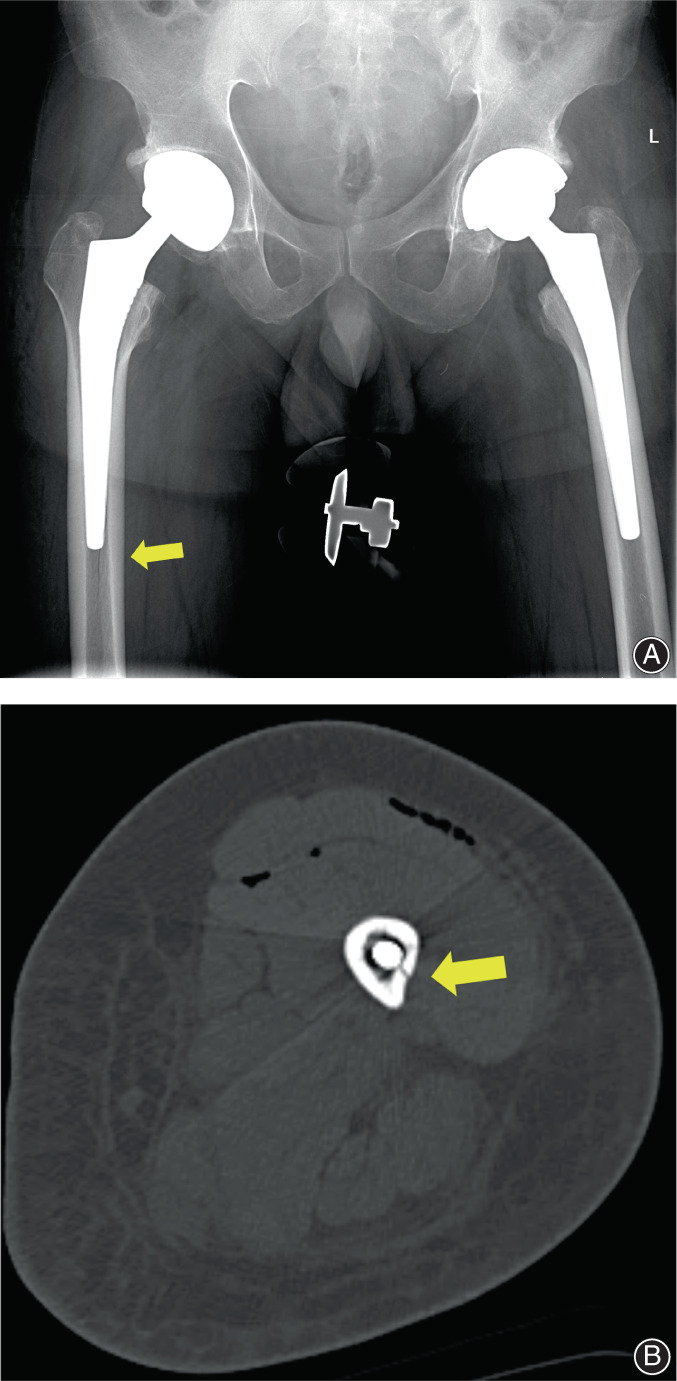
(A) A 52‐year‐old male underwent THA due to ONFH. Postoperative radiograph showed a longitudinal extended fracture at the distal side of the right femur. The fracture healed 3 months postoperatively without reoperation. (B) A 43‐year‐old female underwent THA due to DDH. Postoperative CT showed a fracture of the distal femur around the modular prosthesis. Fracture healing was observed at the 3‐month postoperative follow‐up without reoperation
